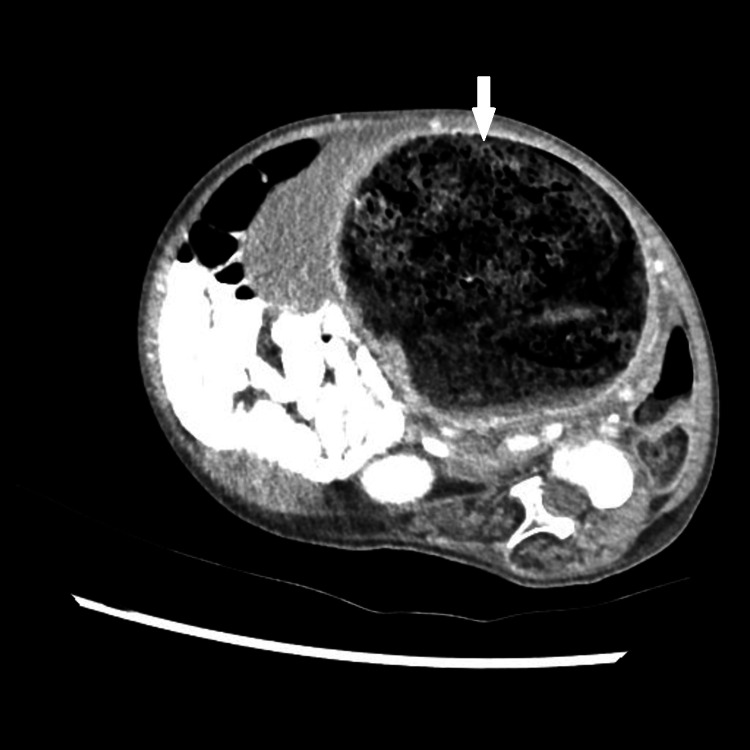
CT scan showing a large fecaloma (white arrowhead). CT: computed tomography
